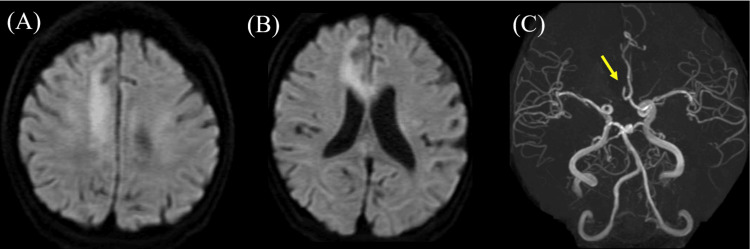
The result of brain MRI and MRA. (A, B) Diffusion-weighted brain MRI showing hyperintensity in the right frontal lobe convexity and corpus callosum. (C) MRA showing occlusion of the right anterior cerebral artery. MRI, magnetic resonance imaging; MRA, magnetic resonance angiography
